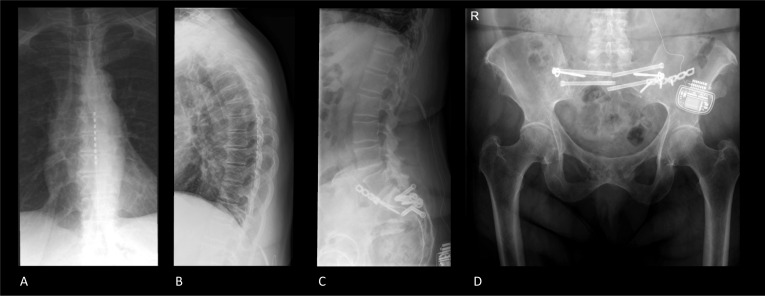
Radiographs of the thoracic- and lumbar spine and pelvic region of an FBSS patient. A The spinal cord stimulation electrode projects centrally at the level of Th8–Th10 on the anterior–posterior view. B The lateral view shows that the electrode projects over the posterior one-third of the spinal canal at levels Th8–Th10. C, D The internalized pulse generator can be observed projecting over the left gluteal region. Extension cables can be observed between the internalized pulse generator and the spinal cord stimulation electrode. Osteosynthesis materials can be observed projecting over the sacroiliac joint bilaterally and the lumbosacral joint. The vertebral column shows no anterior or posterior displacement. Degenerative changes in the thoracic and lumbar vertebrae can be observed with anterior and lateral osteophytes
